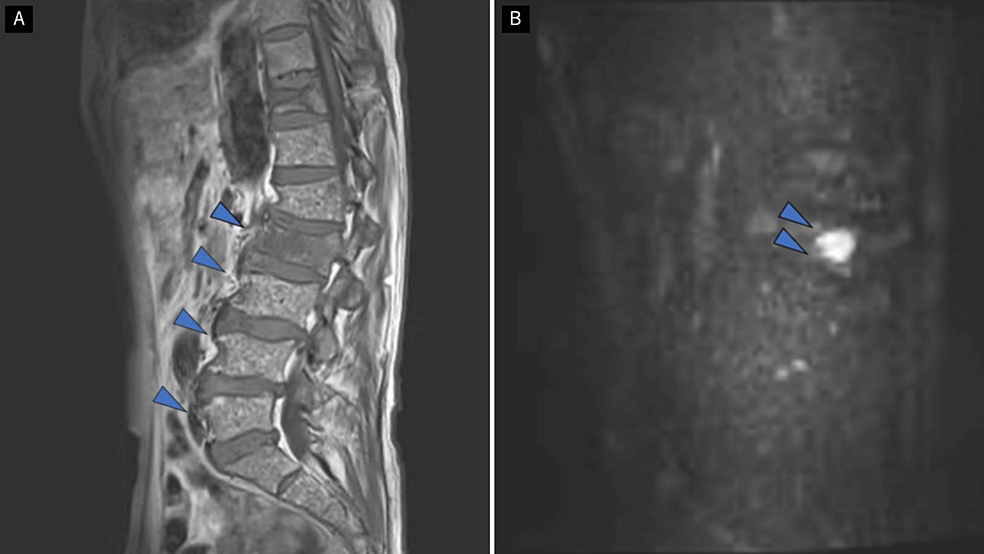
Spine MR imaging (A) T1-weighted MR imaging revealed a "salt-and-pepper pattern" characterized by numerous small foci of low signal intensity (indicated by blue arrows). (B) On high-b value DWI images from Th12 to L2, abnormal high signal intensities were observed in the bone marrow, with a notably strong abnormal signal confirmed at L2 (indicated by blue arrows). DWI: diffusion-weighted imaging
